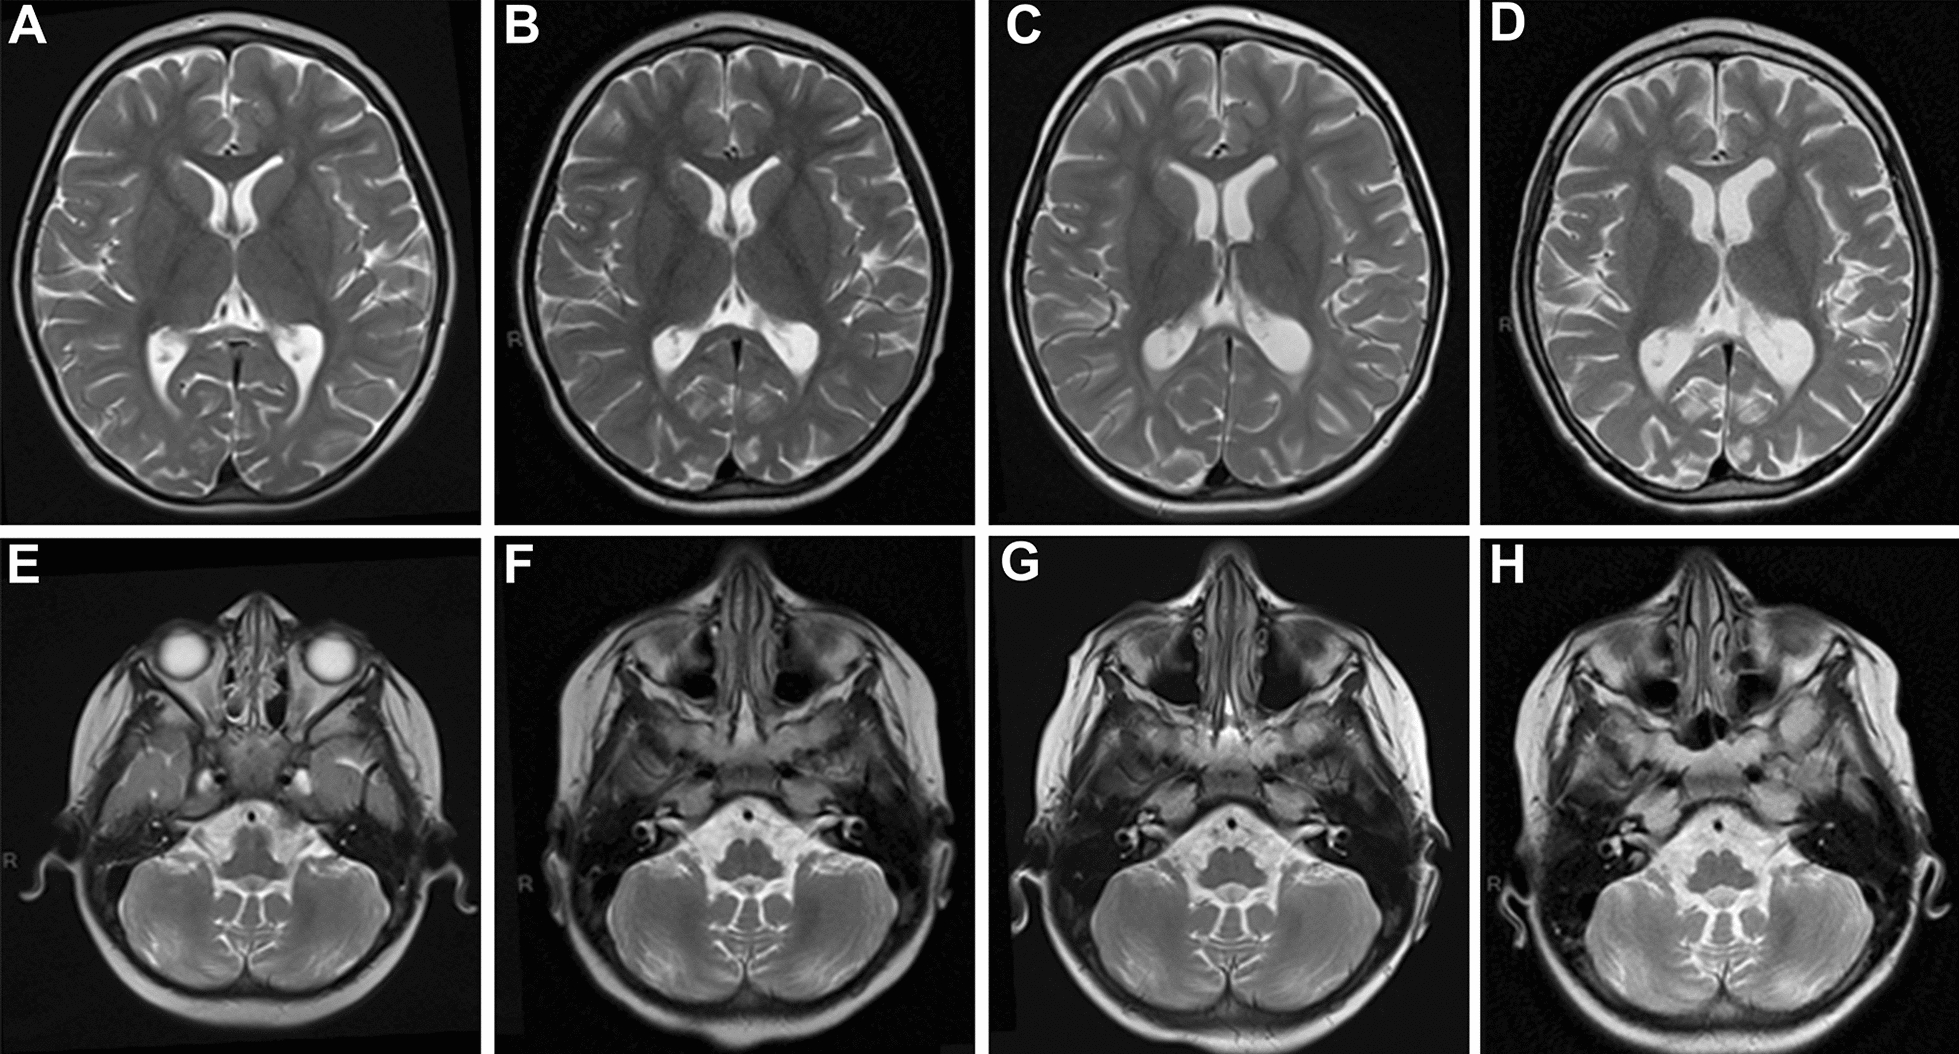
Brain magnetic resonance imaging (MRI) performed at A and E 4 years, B and F 4 years and 5 months, C and G 5 years and 2 months, and D and H 7 years and 6 months of age. T2 axial and T1 sagittal sequences reveal progressive cerebellar and cortical atrophy with slimming of corpus callosum
